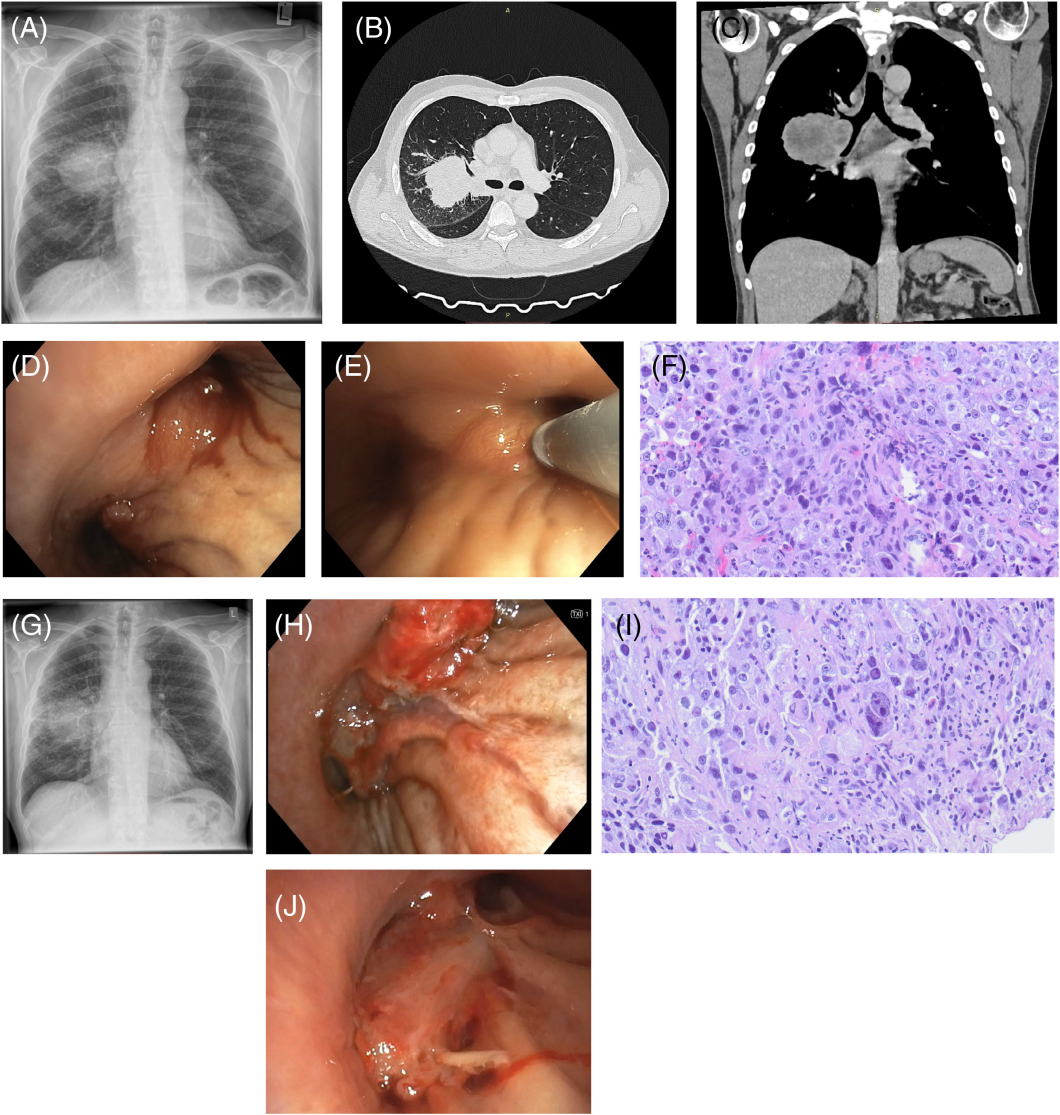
(A) Initial chest x‐ray showing central mass in the right lung. (B, C) CT‐scan showing suspicion of lung cancer in the right lung. (D) Bronchoscopy revealing exophytic lesion with changes in bronchial mucosa. (E) Cryobiopsy of infiltrated bronchial mucosa. (F) HPE of mucosal cryobiopsy showing the presence of adenocarcinoma. (G) Chest x‐ray 10 days after biopsy showing right‐sided pneumonia and slight growth of tumour mass. (H) Repeat bronchoscopy revealing endobronchial growth causing airway obstruction of the right lung. (I) HPE of cryoextraction of the endobronchial growth showing adenocarcinoma. (J) Repeat bronchoscopy showing recurrence of endobronchial tumour growth.
